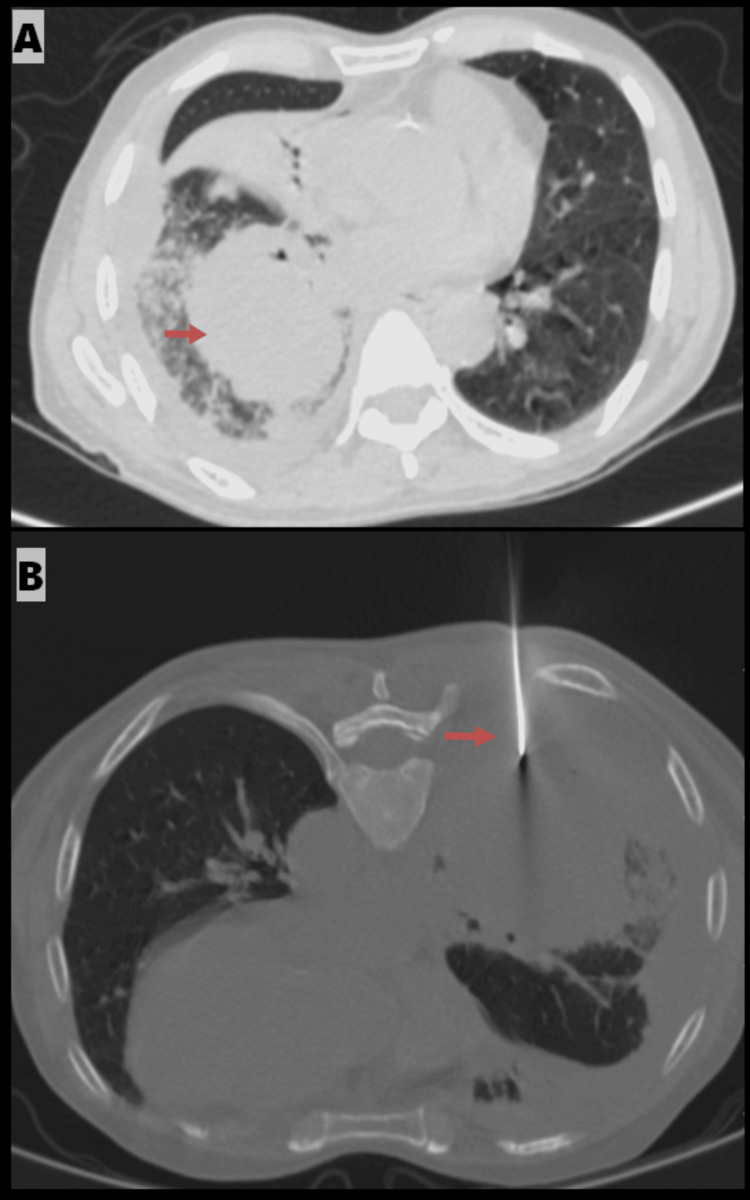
A) Axial contrast-enhanced CT of the chest showing a well-defined soft tissue lesion with irregular lobulated and spiculated margins in the right lower lobe, abutting the mediastinal pleura and pericardial surface, with loss of the fat plane and invasion of the right pulmonary vein (indicated by an arrow). B) Prone axial CT in the mediastinal window demonstrating the biopsy needle within the mass in the right lower lobe (indicated by an arrow).
